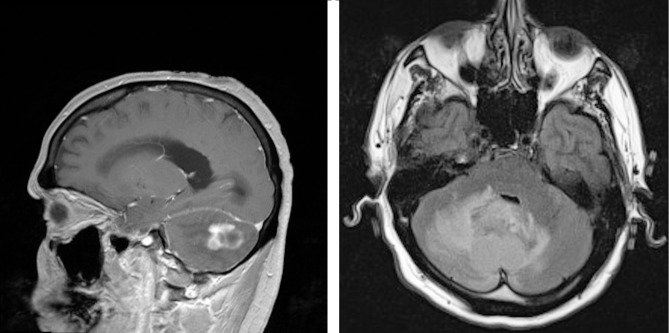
MRI of the brain revealed a 2.6 cm cerebellar lesion.
